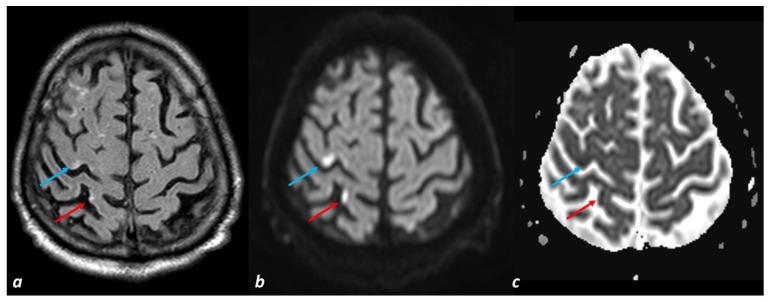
Acute cortical microinfarctions (red and blue arrows) on MRI (axial FLAIR sequence in (a), DWI sequence in (b) and ADC map in (c) in a patient with CAA-related inflammation.
